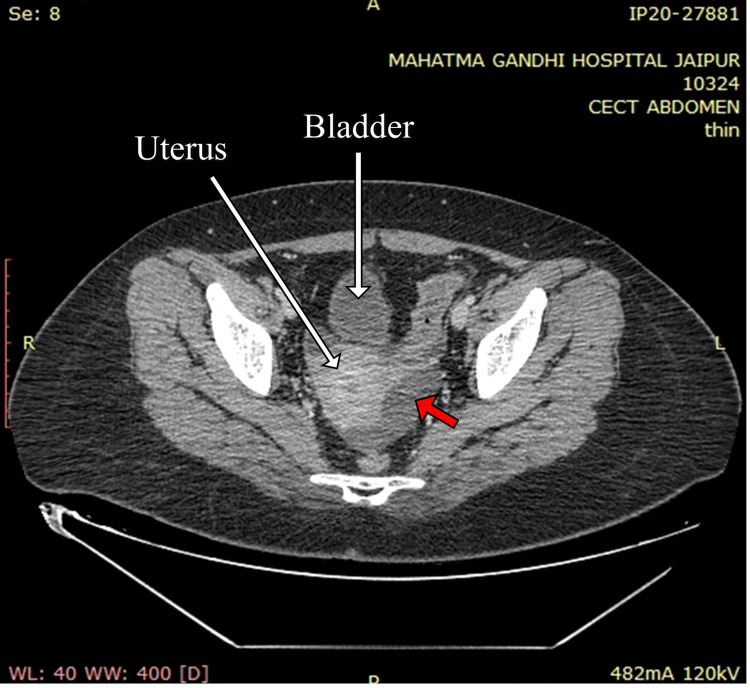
Contrast-enhanced CT of the abdomen (axial section) showing free fluid in the pelvis and ascites (solid red arrow).
